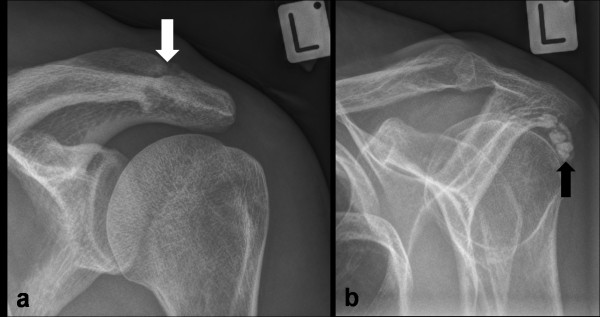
Shoulder x-ray images of ACJ pathology and rotator cuff calcification. a) AP x-ray view in external rotation showing degenerative acromioclavicular joint changes (white arrow); b) outlet view showing calcification in line with the infraspinatus tendon (black arrow).
